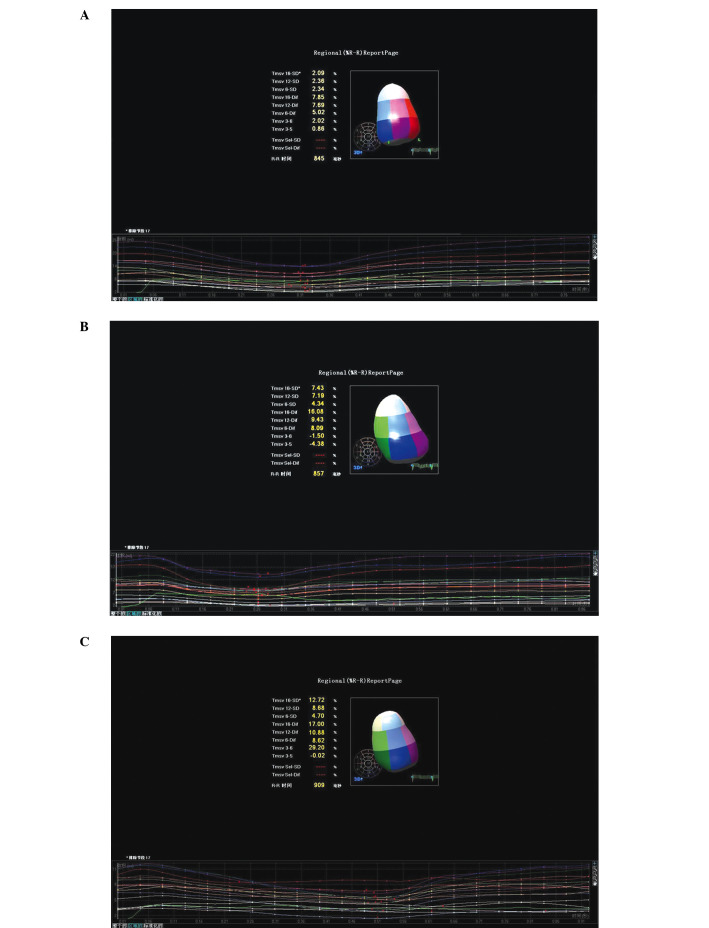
Real-time three-dimensional echocardiography (RT3DE) 17 regional left venrticular (LV) volume-time curves (VTCs) for one patient. (A) VTCs in one patient from the AAI pacing mode. The Tmsv_16_-SD% and Tmsv_16_-Dif% of LV dyssynchrony indices were 2.09 and 7.85%, respectively. (B) VTCs in the same patient from the DDD pacing mode. The Tmsv_16_-SD% and Tmsv_16_-Dif% of LV dyssynchrony indices were 7.43 and 16.08%, respectively. (C) VTCs in the same patient from the VVI pacing mode. The Tmsv_16_-SD% and Tmsv_16_-Dif% of LV dyssynchrony indices were 12.72 and 17.0%, respectively.
